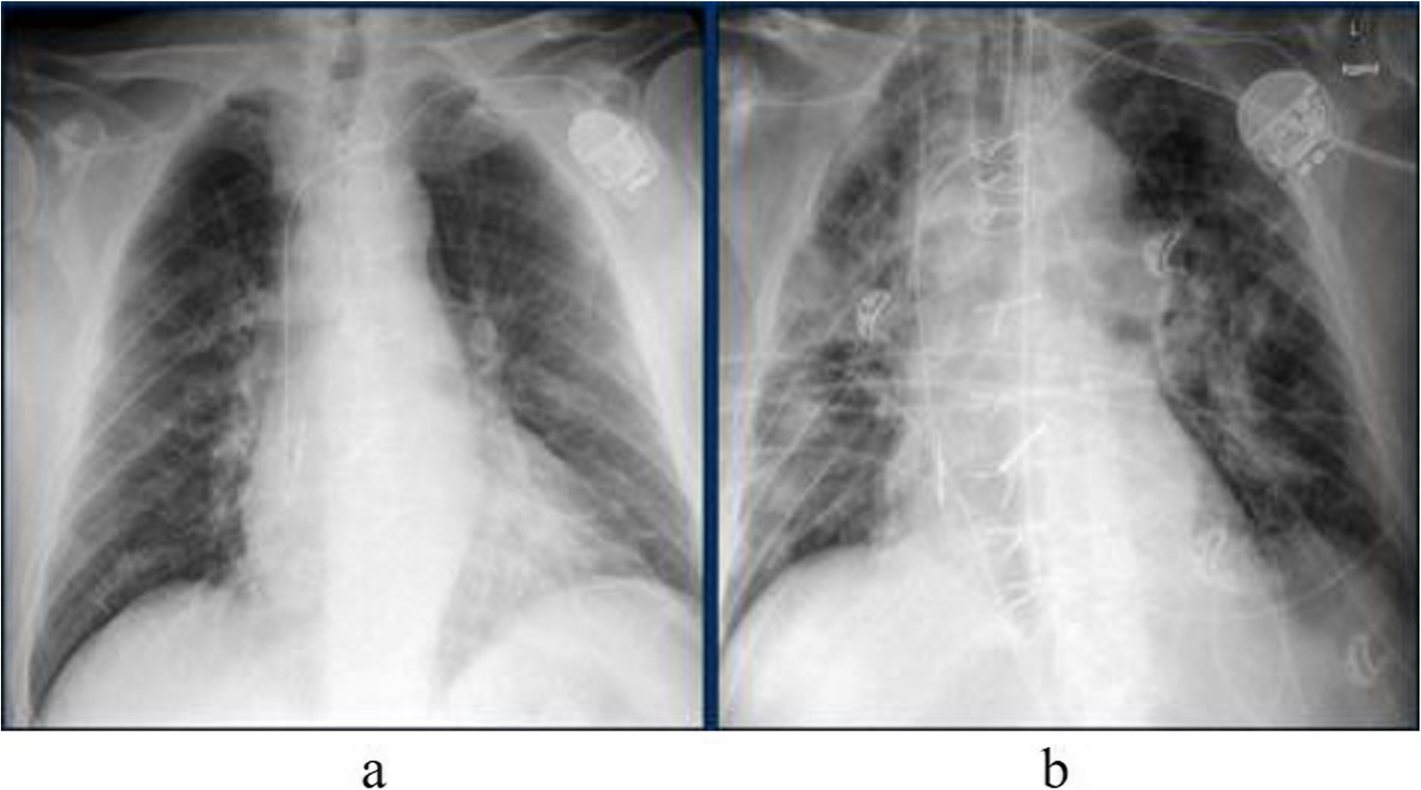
X-rays of a patient with COVID-19. a Normal; b After 4 days with bilateral consolidations [26]
